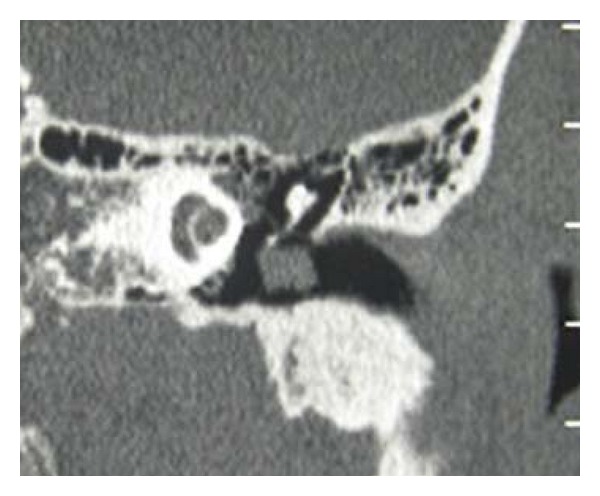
Computed tomography scanning without means of contrast. A 3 mm soft tissue mass is localized in the superior part of ear drum in correspondence of malleus with no present signs of erosion. Middle ear cavity and the other ossicles appeared normal.
